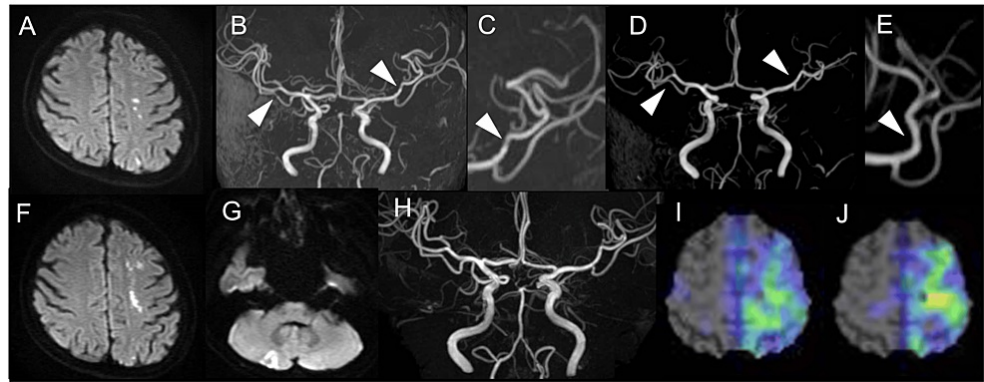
Imaging findings of the two episodes of cerebral infarction and subsequent hospitalization (A) Diffusion-weighted images (DWI) at index stroke showing multiple acute ischemic lesions in the left middle cerebral artery (MCA) watershed area. (B) Magnetic resonance angiography (MRA) at index stroke showing mild stenosis of the bilateral MCA. (C) Magnified image of the left MCA in part B. (D) MRA at nine days after index stroke onset showing improvement of the multiple stenosis. € Magnified image of the left MCA in part D. (F) DWI at the time of the subsequent stroke showing recurrence in the same watershed areas. (G) DWI at the time of the subsequent stroke showing a new infarction in the right cerebellar area. (H) Visible stenosis not evident in MRA at the time of the subsequent stroke. (I) Iodine-123-labeled N-isopropyl-p-iodo-amphetamine Single-photon emission-computed tomography (IMP-SPECT) images at the time of the subsequent stroke showing cerebral blood flow (CBF) reduction in the left MCA area. (J) SPECT images two months after the subsequent stroke. No changes to CBF reduction were observed.
